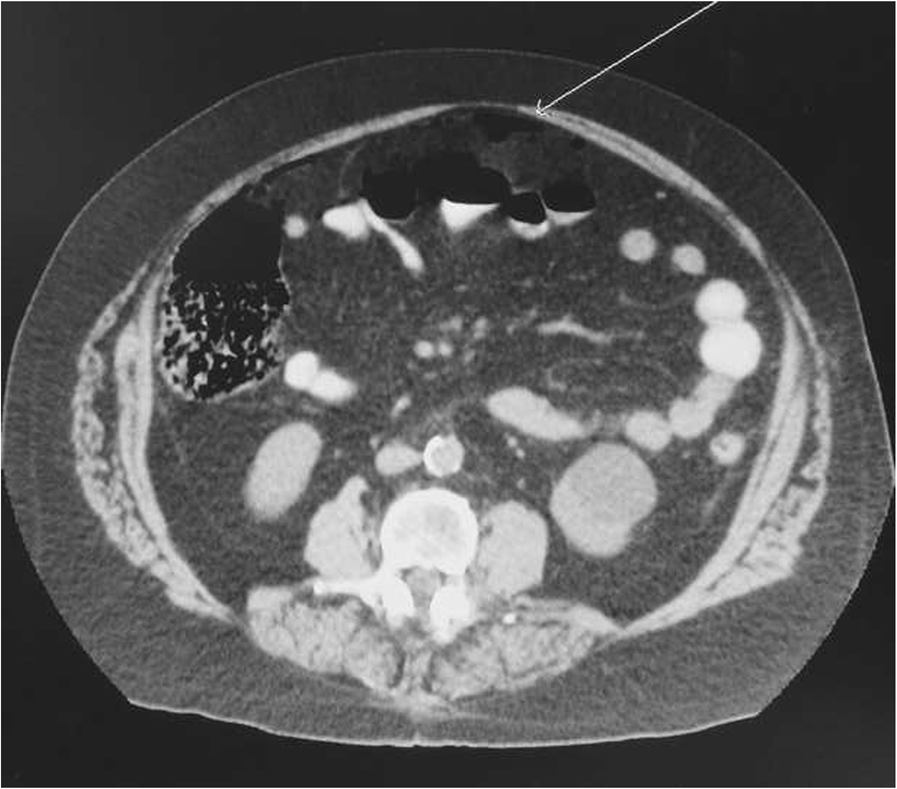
CT scan of the abdomen. Pneumoperitoneum identified on CT scan of the abdomen
